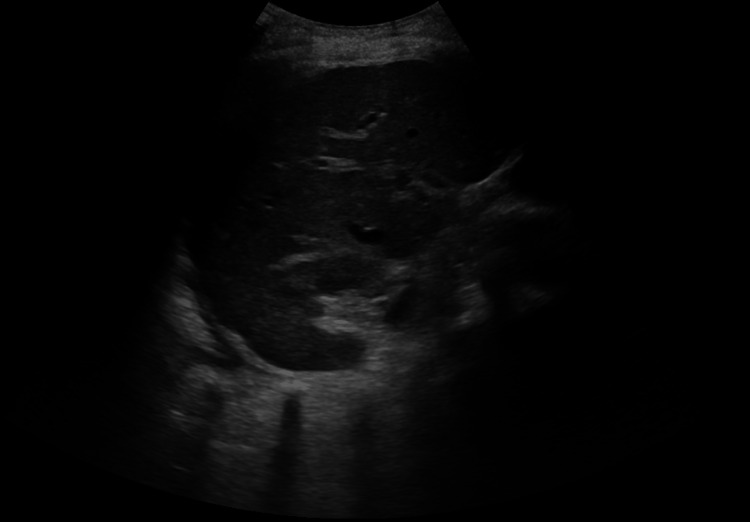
Liver ultrasound demonstrating normal echogenicity of liver without biliary dilatation
